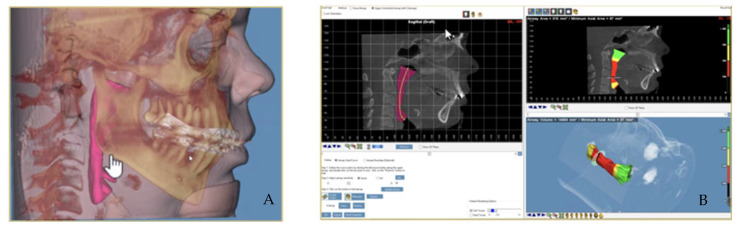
Airway measurement with CBCT: anatomic boundaries of the upper pharyngeal airway (pink) (A). Segmentation of the upper airways: nasopharynx (green), oropharynx (red) and hypopharynx (lower yellow) (B).
